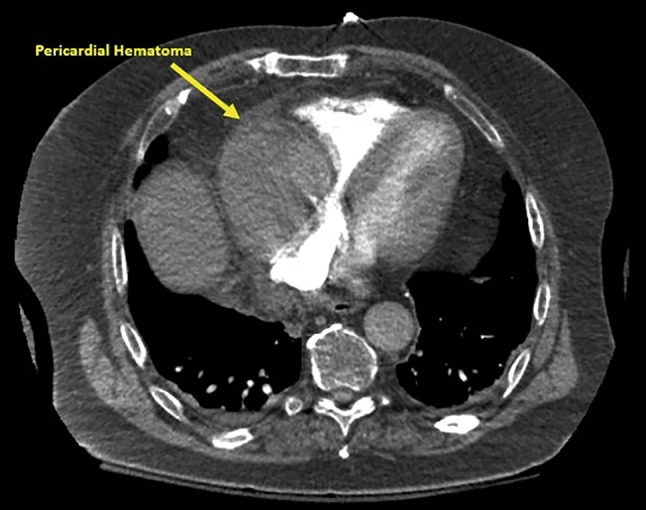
Computed tomography finding
